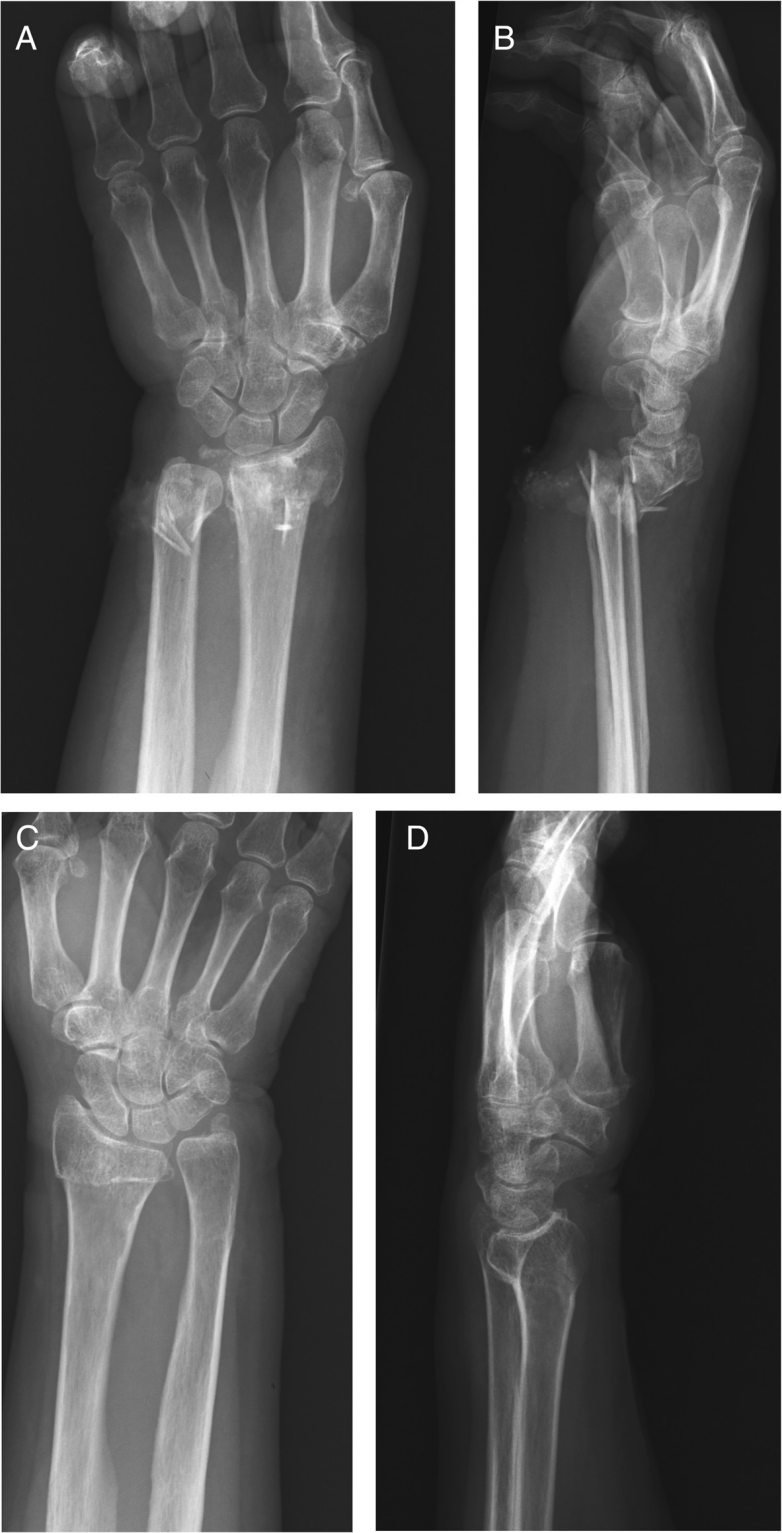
Radiographs showed bilateral distal radius fractures. According to the Arbeitsgemeinschaft für Osteosynthesefragen (AO) classification, right side is A3 and left side is A1. A: Right side, A-P view. B: Right side, lateral view. C: Left side, A-P view. D: Left side, lateral view.
